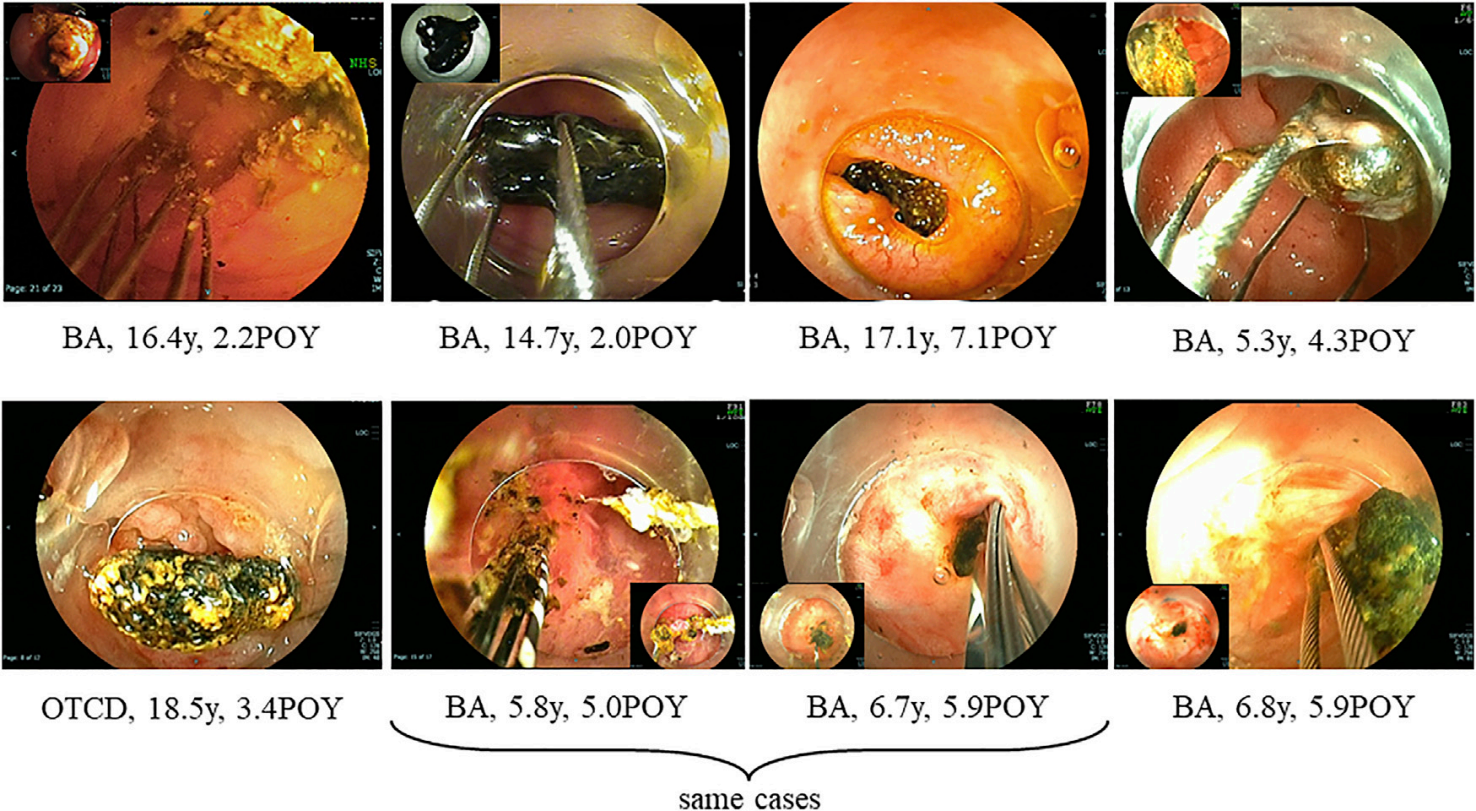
Types of hepatolithiasis diagnosed by computed tomography scan before treatment for post-transplant anastomotic biliary strictures. BA; biliary atresia, POY; post-operative year, OTCD; ornithine transcarbamylase deficiency.
